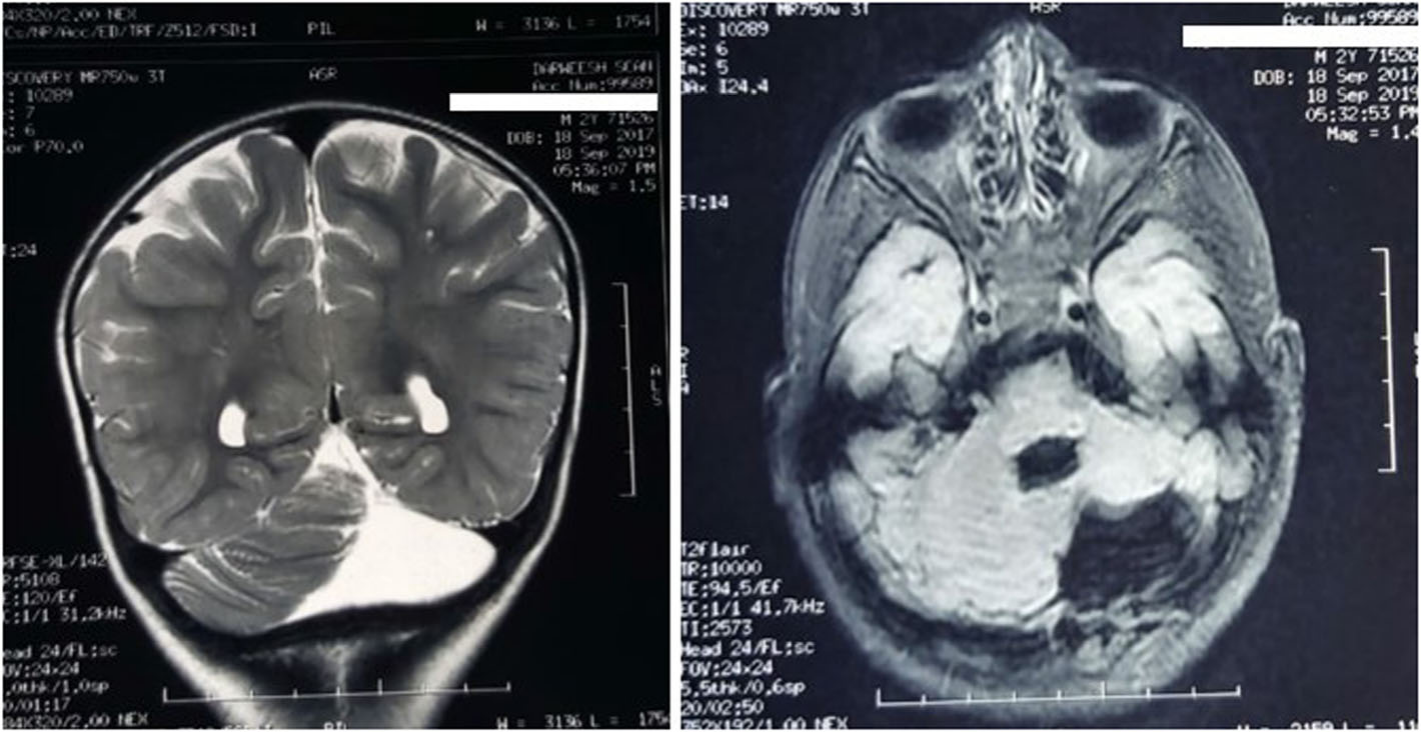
Magnetic resonance imaging of the brain revealed hypoplasia of the antero-superior compartment and total aplasia of the postero-inferior compartment of the left cerebellar hemisphere
